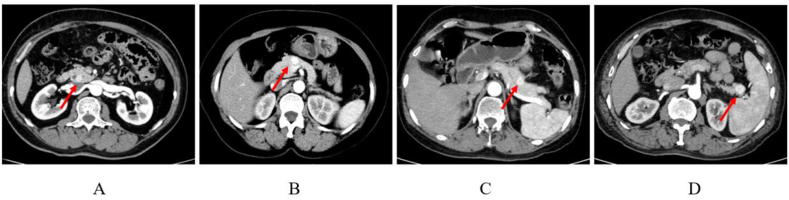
Enhanced computed tomography images of the abdomen. Arrow shows the hyperintense insulinoma in the pancreatic head (A), neck (B), body (C), and tail (D).
